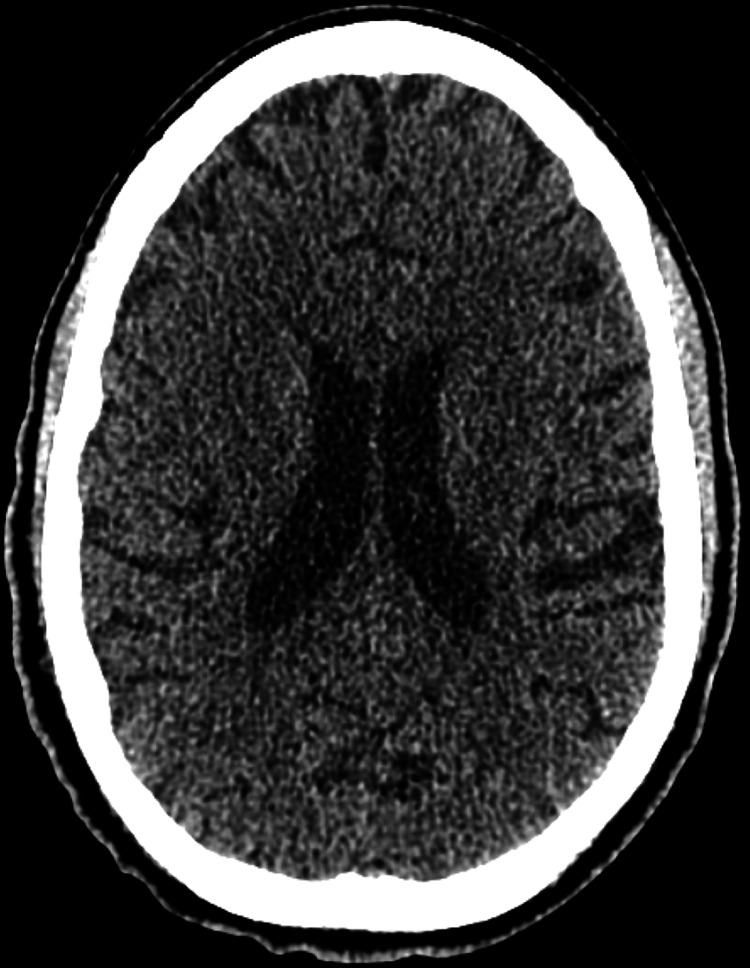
CT scan without intravenous contrast of the head showing no hemorrhage or acute large territorial infarct. The ventricles and basal cisterns are patent. There is no hydrocephalus or midline shift. Overall, no acute intracranial abnormality.
